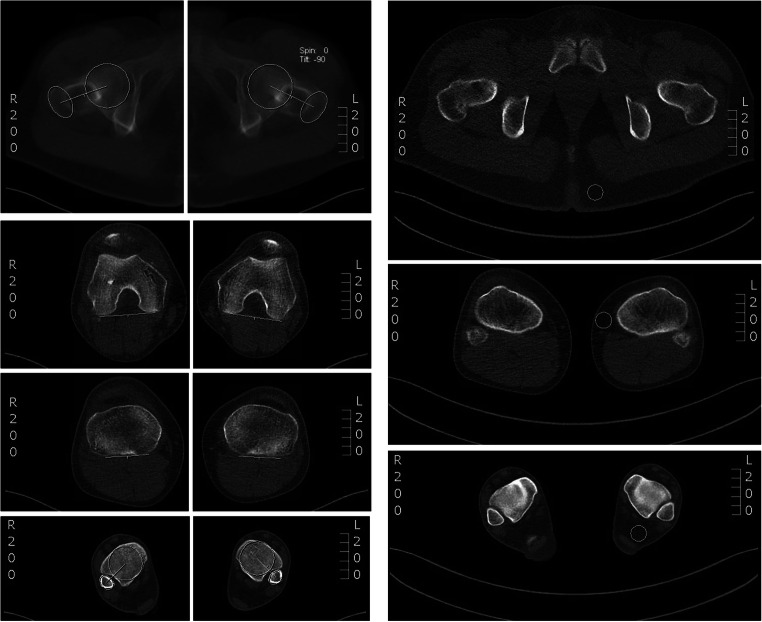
CT torsion measurement of the lower limb using a technique modified from Waidelich et al [20] and measurement of image noise in fatty tissue at standardized anatomical landmarks
